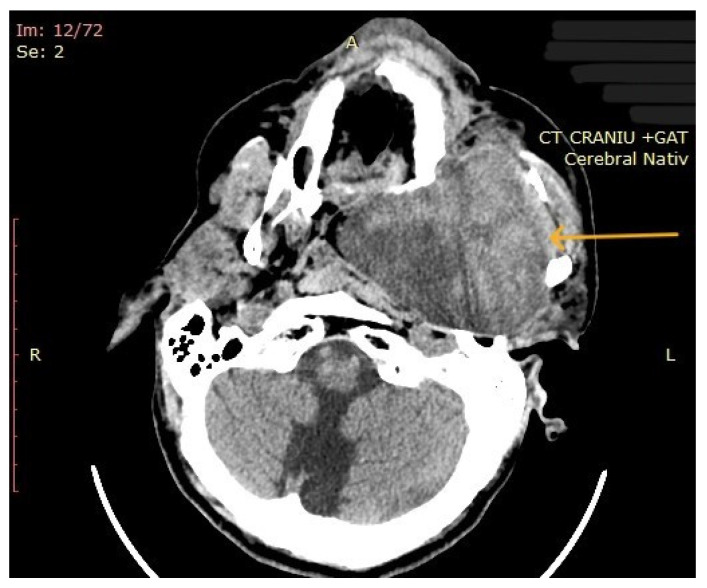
CT imaging: extension of the mass towards the nasopharynx and oro- and hypopharynx (the arrow represents the tumor mass).
